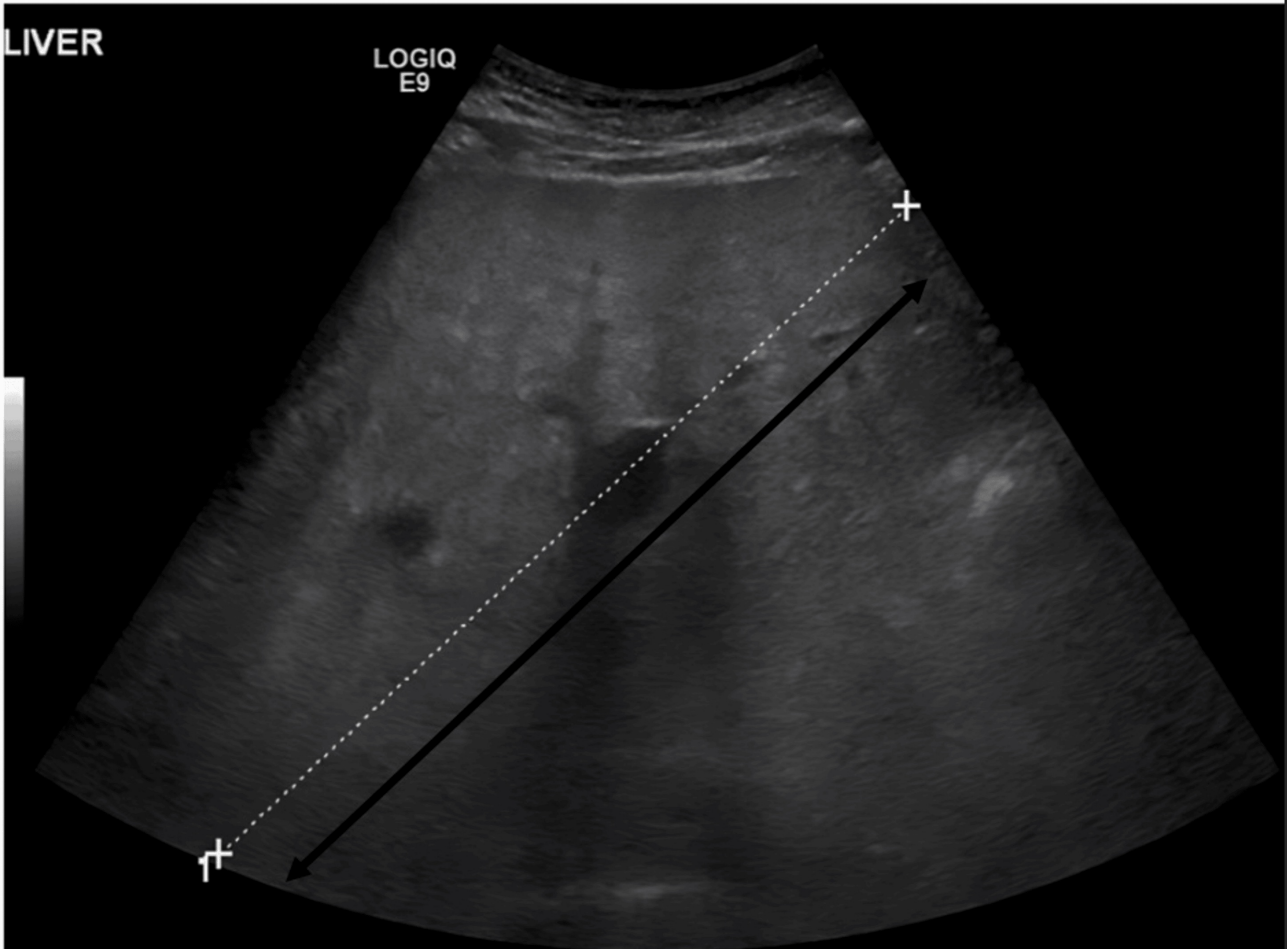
Abdominal ultrasound showing hepatomegaly measuring approximately 19 cm
